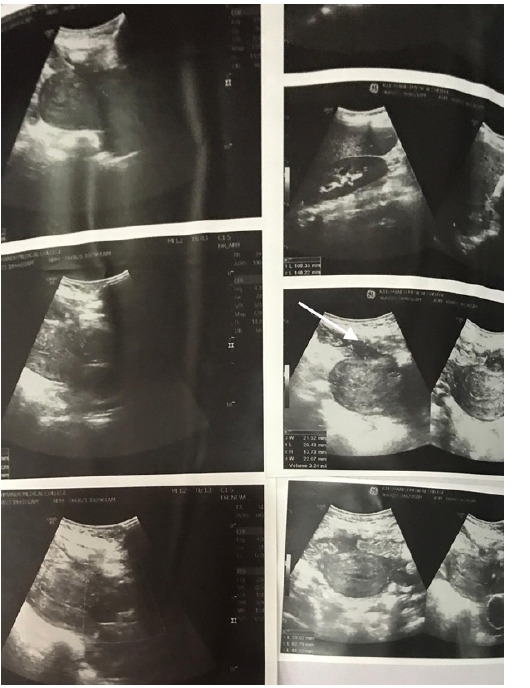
Ultrasonographic images after 9 days showing minimal uterovesical collection.
